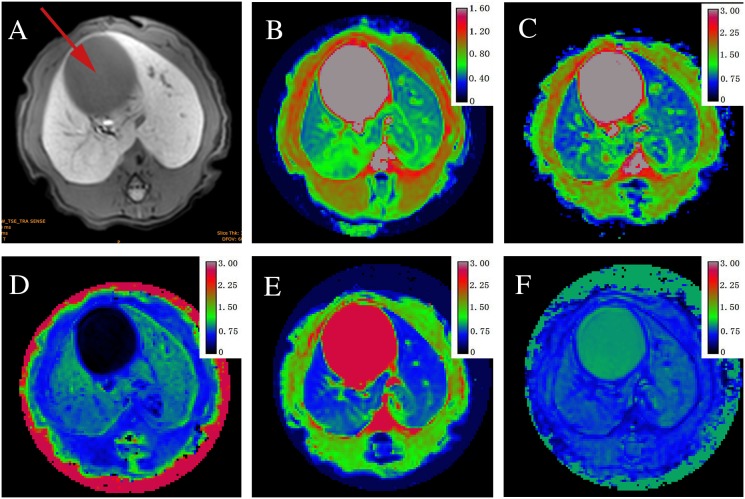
Axial MR images in sequence from a stage F2 fibrosis induced by biliary duct ligation (BDL) (A) T1-weighted image, red arrow = dilated biliary duct; (B) mean values (0.88×10^−3^ mm^2^/s) shown by a map of apparent diffusion coefficients (ADC); (C) mean values (0.99×10^−3^ mm^2^/s) shown by the D_app_ map; (D) mean values (1.07) shown by the K_app_ map; (E) mean values (0.68×10^−3^ mm^2^/s) shown by DDC map; (F) mean values α (0.64) shown by map.
